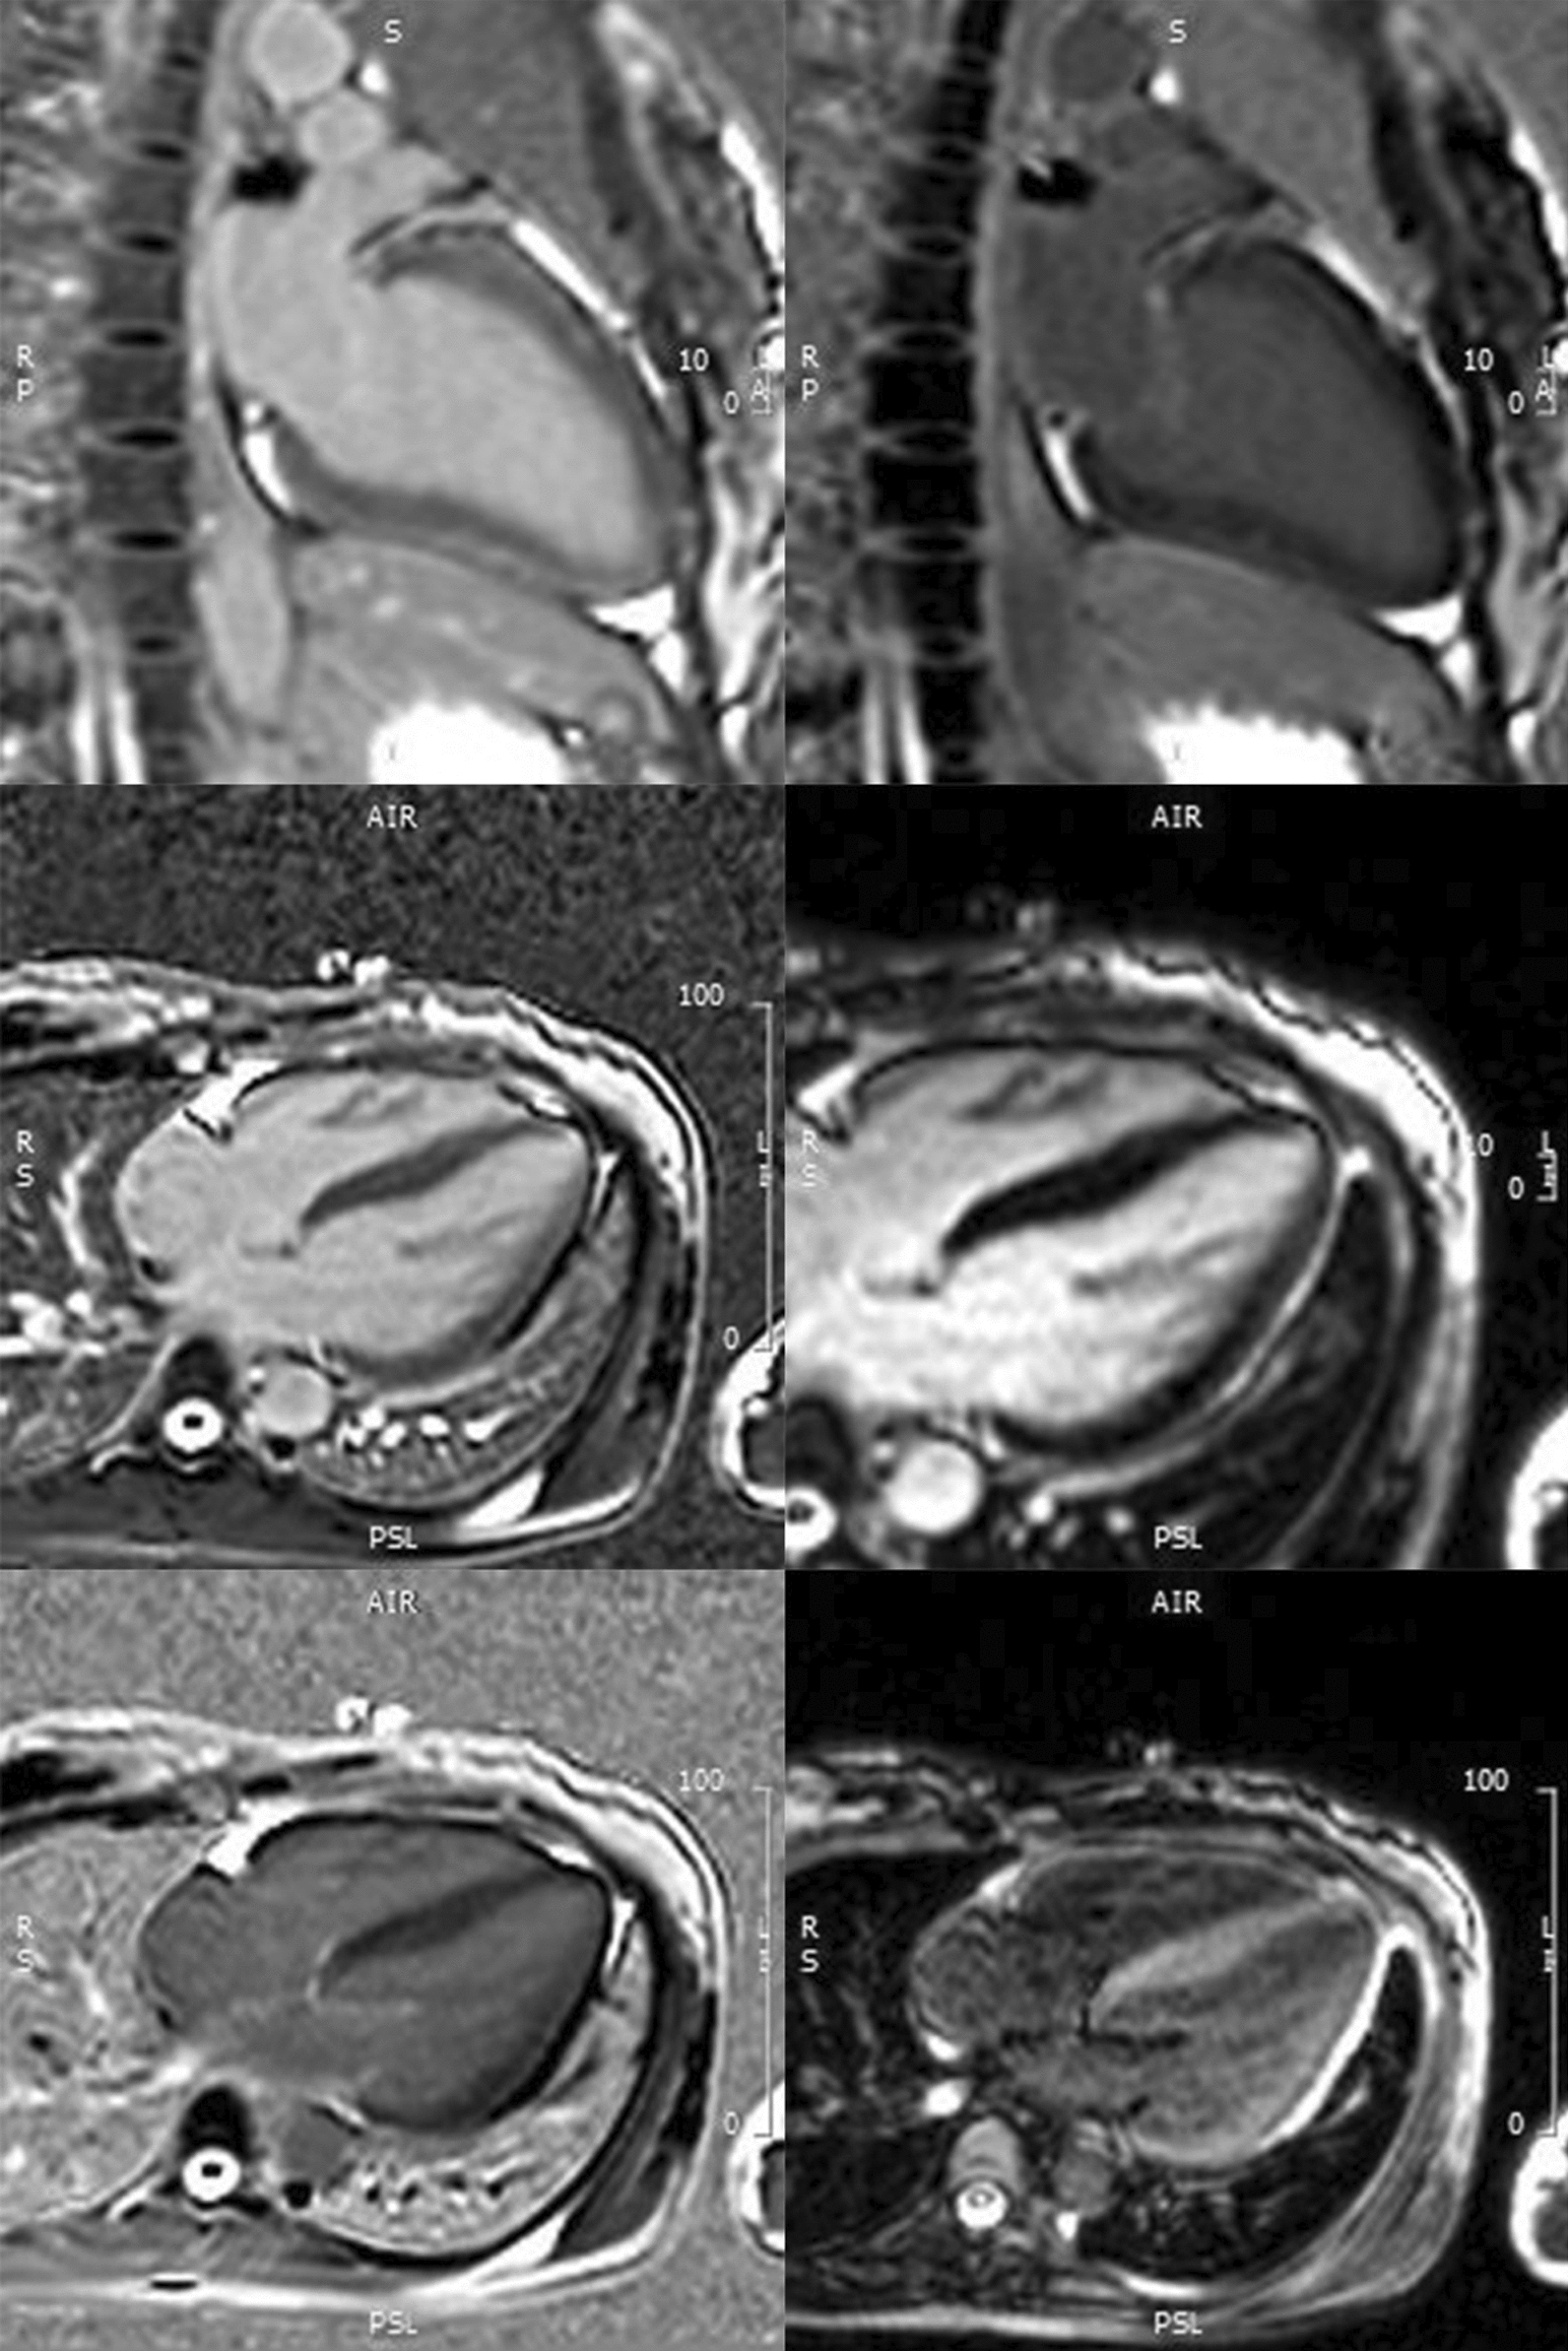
Cardiac magnetic resonance imaging showing subepicardial fibrosis at basal-mid anterior, mid lateral, basal inferior; intramyocardial fibrosis at mid septal; patchy fibrosis at mid posterior right-left ventricular junction and apico-inferoseptal wall consistent with myocarditis
